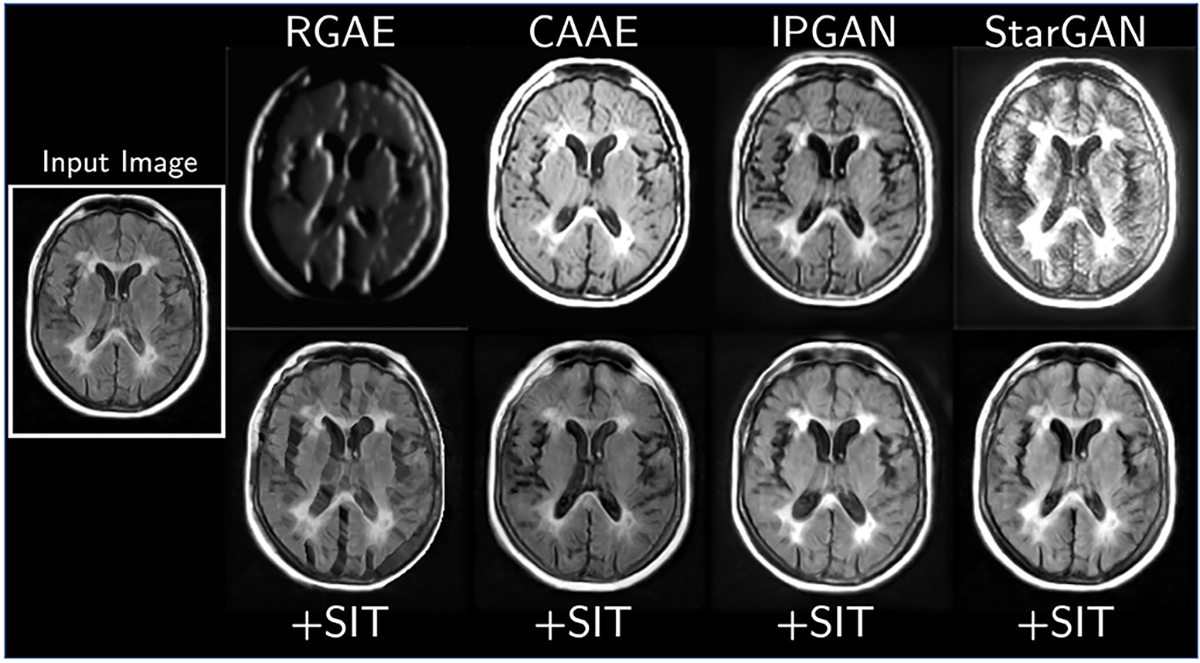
A scan from MRI-GENIE translated to a different age (originally 67 years old, translated to 82 years old) using four unconstrained models *(top row)* and their SIT variants *(bottom row)*.
